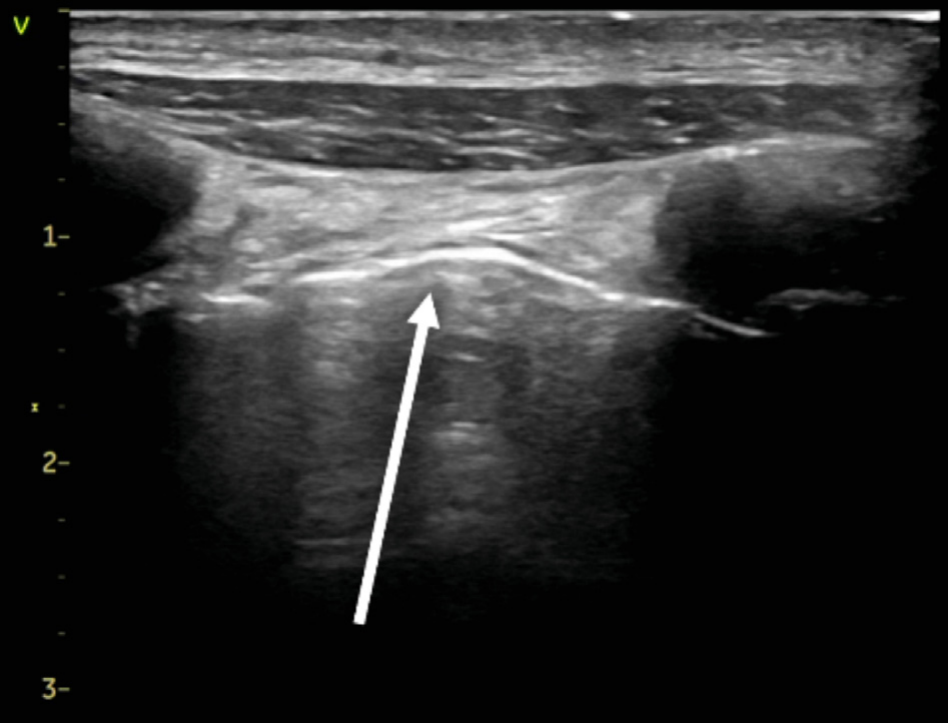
Lung ultrasonography showing sliding signs (arrow).
